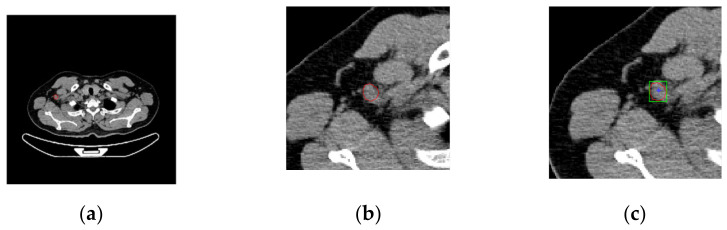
Labeling of lesion segmentation. (a) Delineated lesion on the CT image. (b) Local enlarged view of delineated focus. (c) Divide the lesion with a rectangular box.
